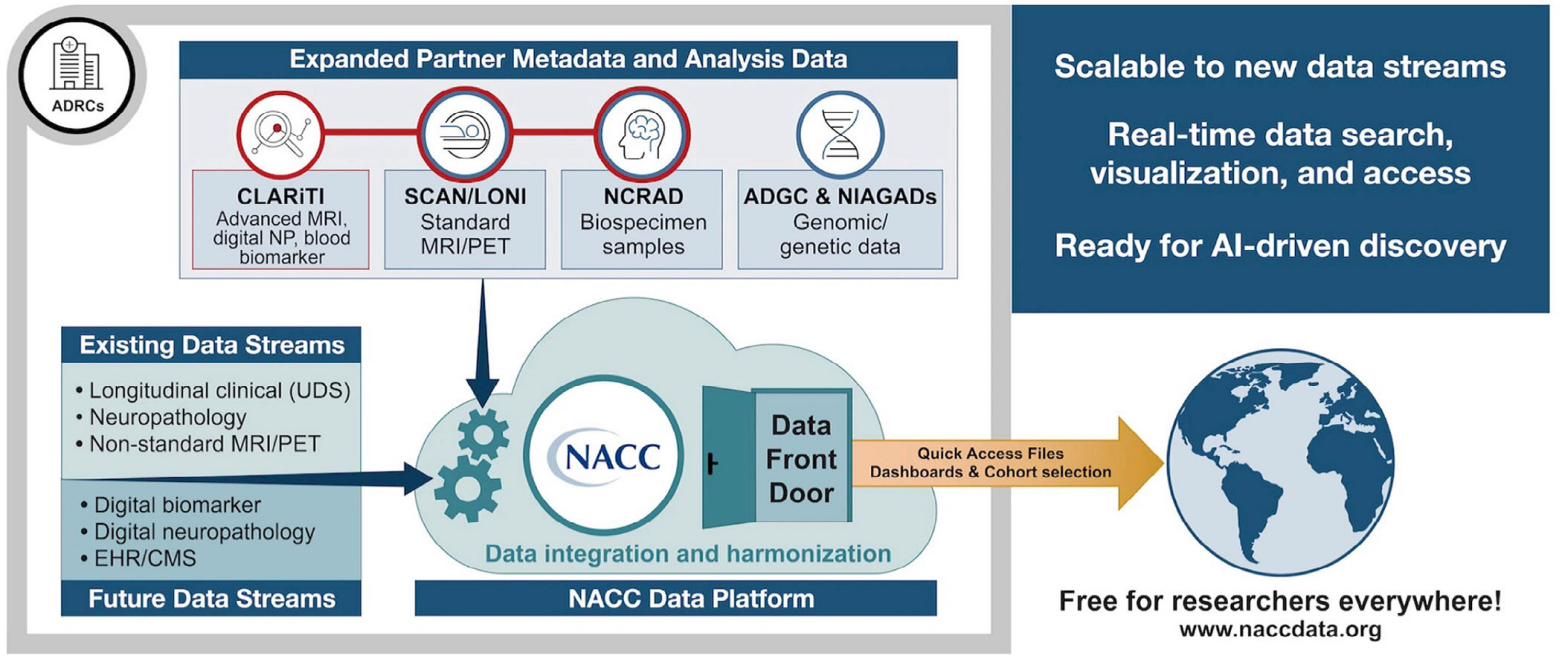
Summary of ADRC infrastructure and data streams relevant for wide‐scale data sharing. ADGC, Alzheimer's Disease Genetics Consortium; ADRC, Alzheimer's Disease Research Center; AI, artificial intelligence; CLARiTI, ADRC Consortium for Clarity in ADRD Research Through Imaging; CMS, Centers for Medicare and Medicaid Services; EHR, electronic health record; LONI, Laboratory of Neuroimaging; MRI, magnetic resonance imaging; NACC, National Alzheimer's Coordinating Center; NCRAD, National Centralized Repository for Alzheimer's Disease and Related Dementias; NIAGADS, National Institute on Aging Genetics of Alzheimer's Disease Data Storage Site; PET, positron emission tomography; RFA, request for application; SCAN, Standardized, Centralized AD/ADRD Neuroimaging; UDS, Uniform Data Set.
